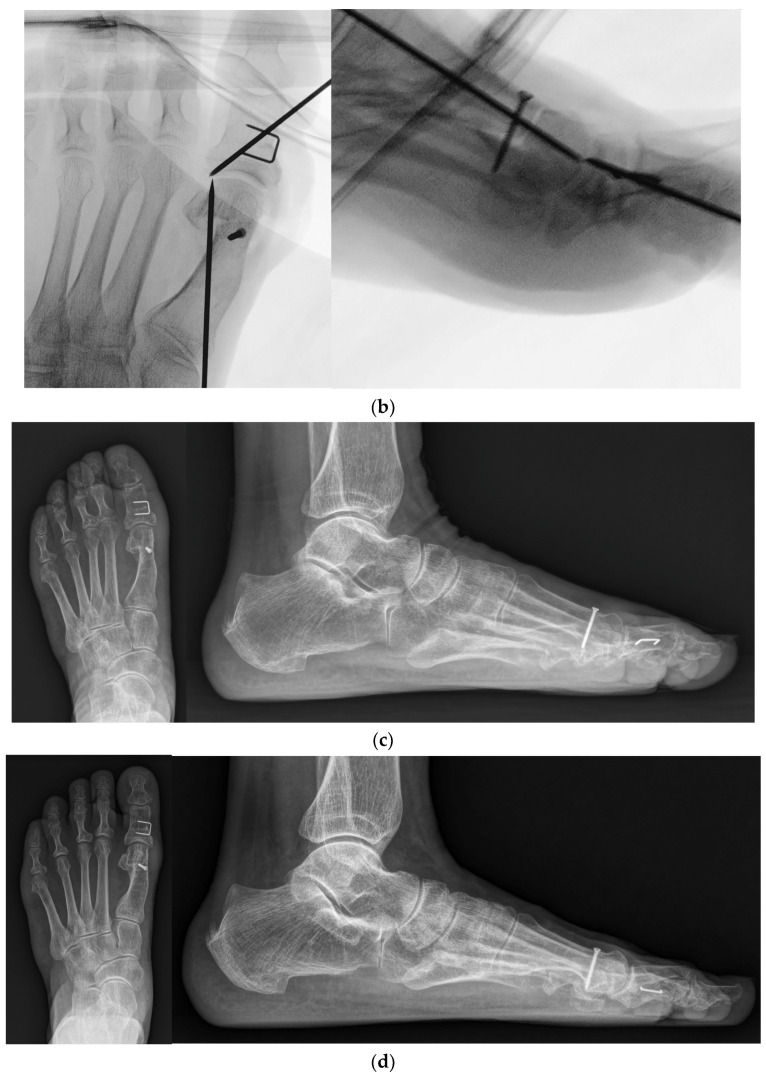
(a) Preoperative standing foot anteroposterior and lateral radiographs; (b) Intraoperative foot anteroposterior and lateral fluoroscopy images; (c) Standing foot anteroposterior and lateral radiographs at 2 months post operation; (d) Standing foot anteroposterior and lateral radiographs at the final follow-up.
